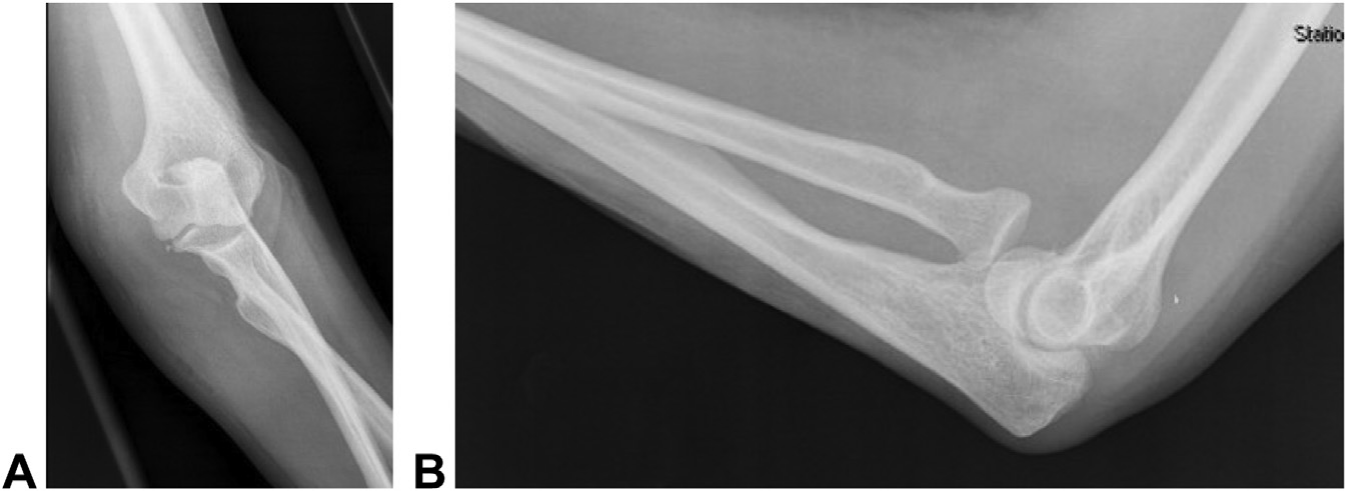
A Anteroposterior and B lateral radiographs at presentation demonstrate anteromedial dislocation of radial head without associated fractures.
